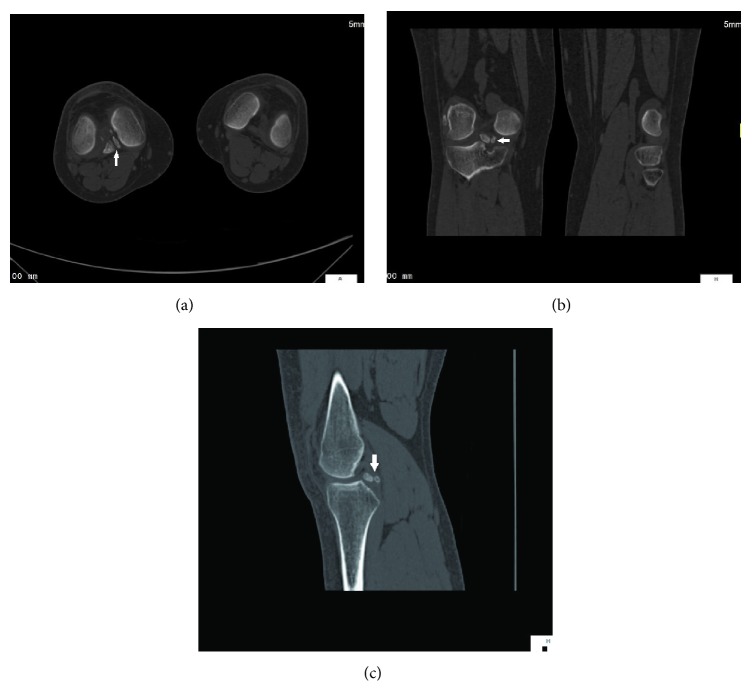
A 21-year young male student presented with chronic pain in the right knee joint for 1-2 years subsequently diagnosed as double meniscal ossicle of the knee. (a) Plain CT axial bone window images show well defined lamellated bone density lesions, two in number (solid arrow) in intra-articular region on medial aspect of right knee. (b) Plain CT coronal bone window images show well defined lamellated bone density lesions, two in number (solid arrow) in intra-articular region on medial aspect of right knee. (c) Plain CT sagittal bone window images show well defined lamellated bone density lesions, two in number (solid arrow) in intra-articular region on medial aspect of right knee.
